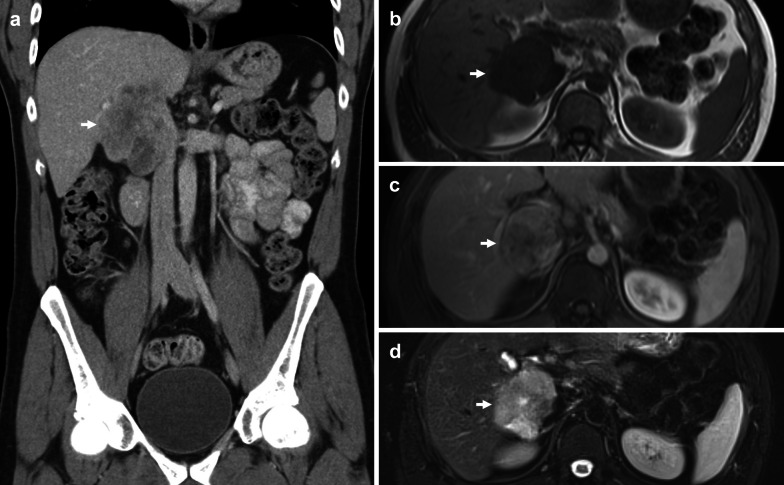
IVC Leiomyosarcoma. a Coronal CT image shows a large lobulated heterogeneously enhancing mass (arrow) between the liver and right kidney. It demonstrates both intra and extraluminal growth. On axial MRI, the lesion (arrows) is (b) homogeneously T1 hypointense to skeletal muscle, (c) enhances heterogeneously, and (d) is heterogeneously T2 hyperintense. Some non-enhancing areas are bright on T2 weighted sequences, suggestive of cystic or necrotic components. MRI also demonstrates a positive embedded organ sign
